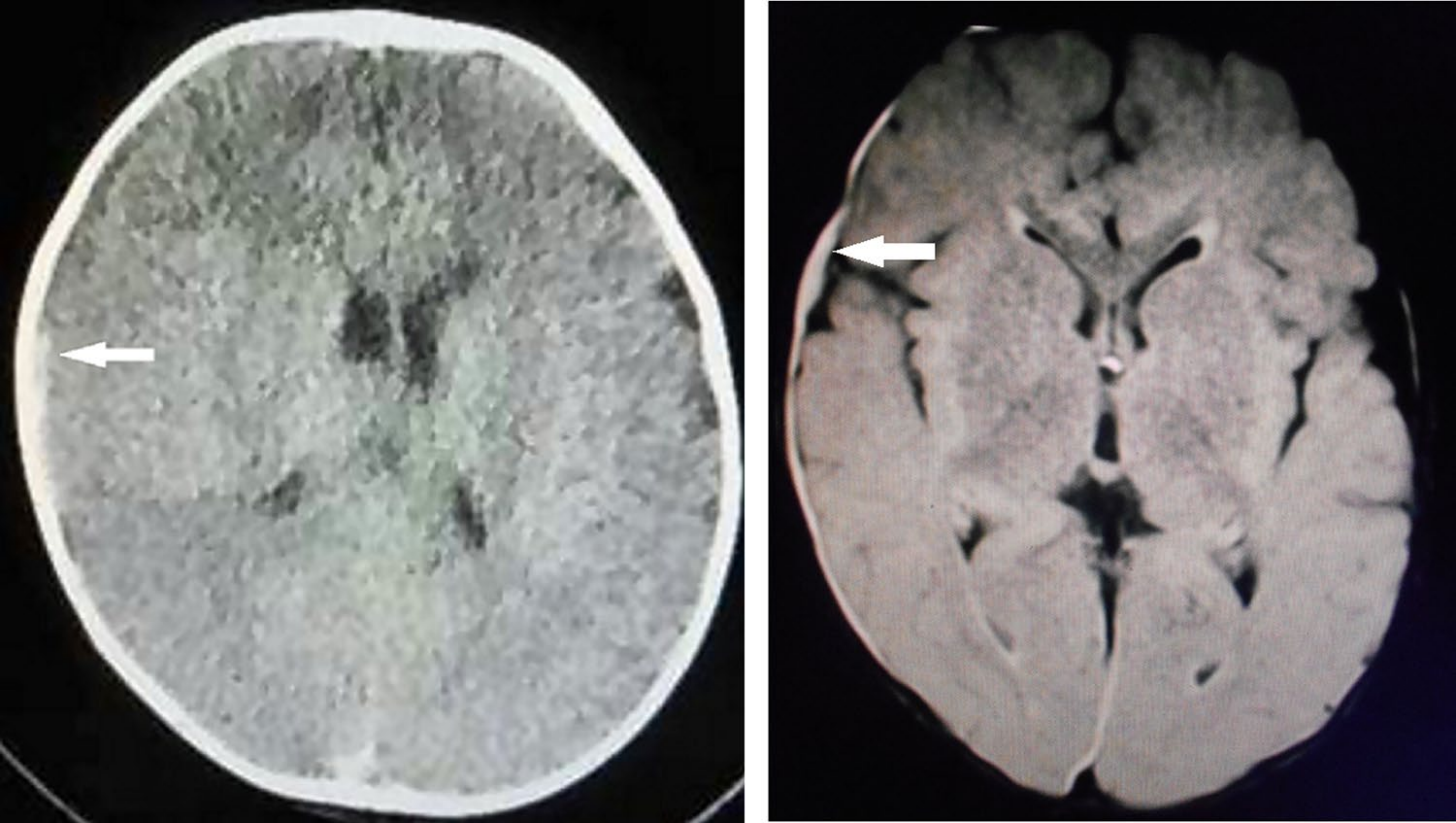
Case 2, male aged 10 months. Left: CT on arrival demonstrating a thin SDH with heterogeneous density on the right side (arrow). Note the low-density sediment formation in the upper part and high- density sediment formation in the lower part. Right: MRI (FLAIR) 3 days after symptom onset demonstrating a thin, film-like, high-intensity SDH on the right side (arrow). No mass effect or parenchymal abnormality was observed on the other sequences (not shown)
